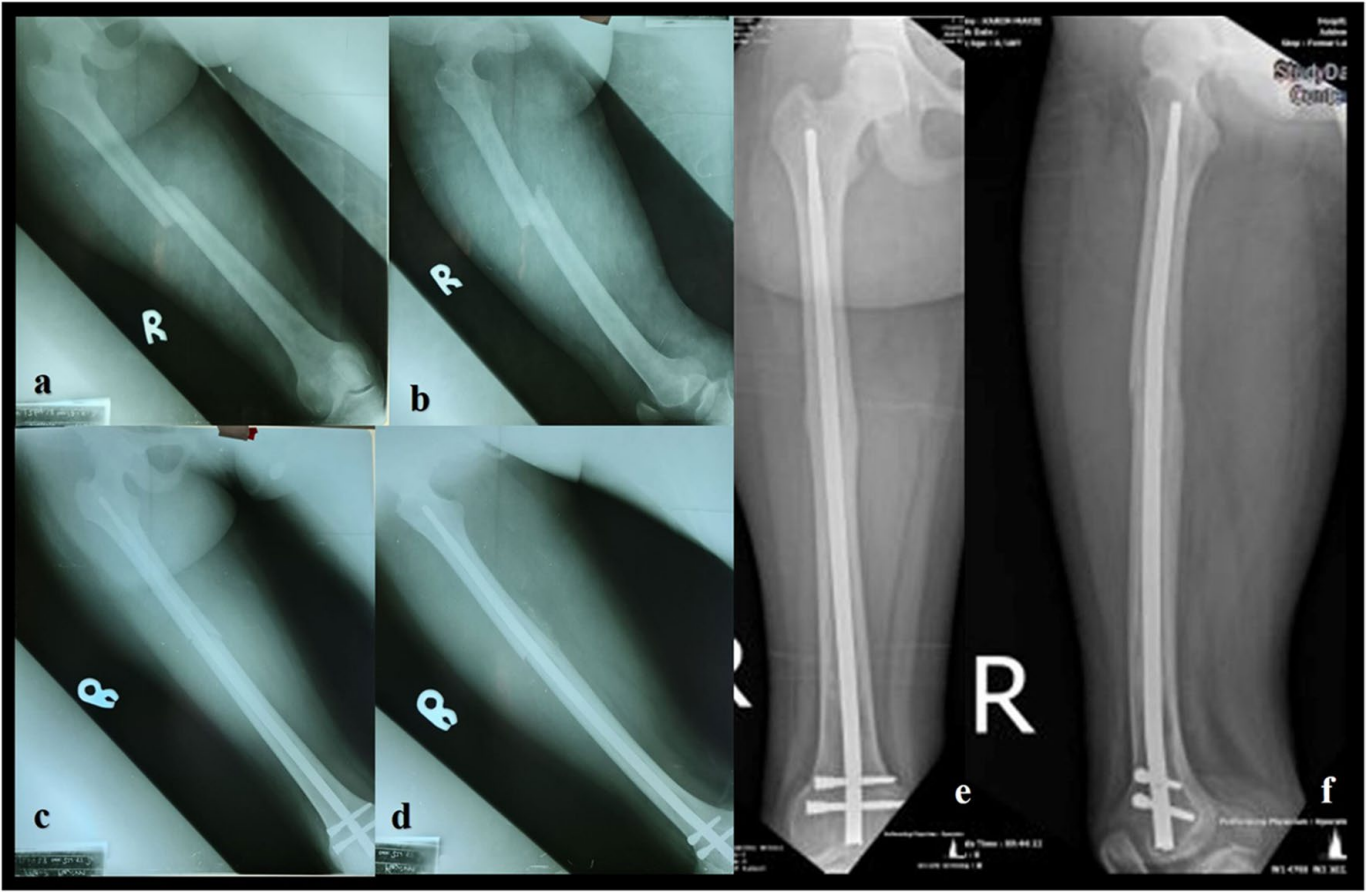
A 40 year old female patient with femoral midshaft fractures treated using SFN®, (a, b) preoperative radiographs, (c, d) postoperative radiographs, (e, f) consolidation at the follow up at 12 months
